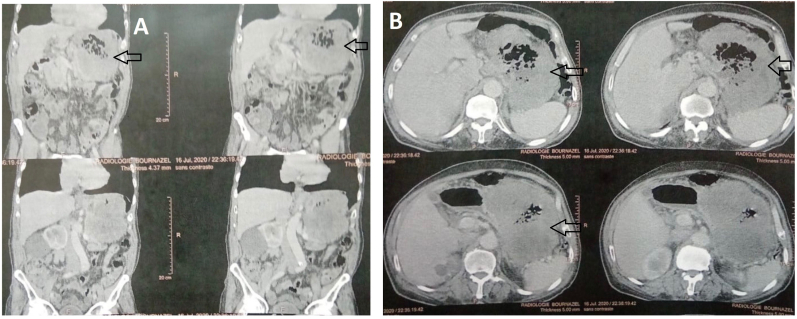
(A, B) Enhanced computed tomography (CT) scan of the abdomen (axial and coronal planes) revealing a large tumor (left arrow) adjacent to greater curvature of the stomach.
